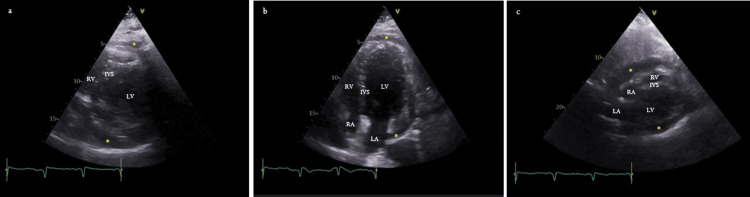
Transthoracic echocardiogram demonstrates a moderate circumferential pericardial effusion (asterisks), measuring 15 mm (a) Parasternal short axis. (b) Apical four-chamber view. (c) Subcostal view. LV: left ventricle, RV: right ventricle, LA: left atrium, RA: right atrium, IVS: interventricular septum
